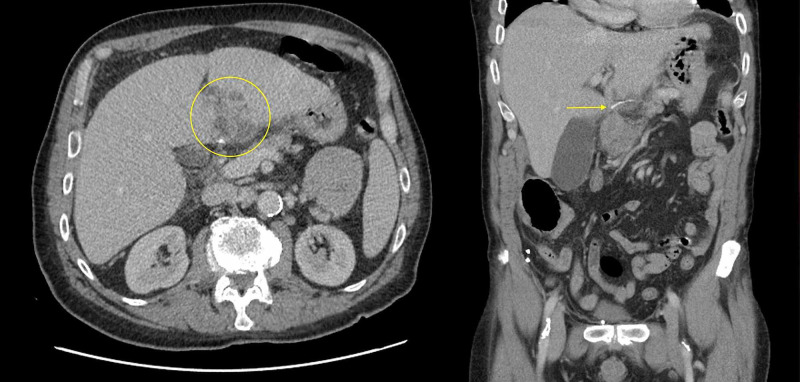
CT scan demonstrating the hepatic abscess with an associated linear density and localised inflammation changes CT - Computed tomography
